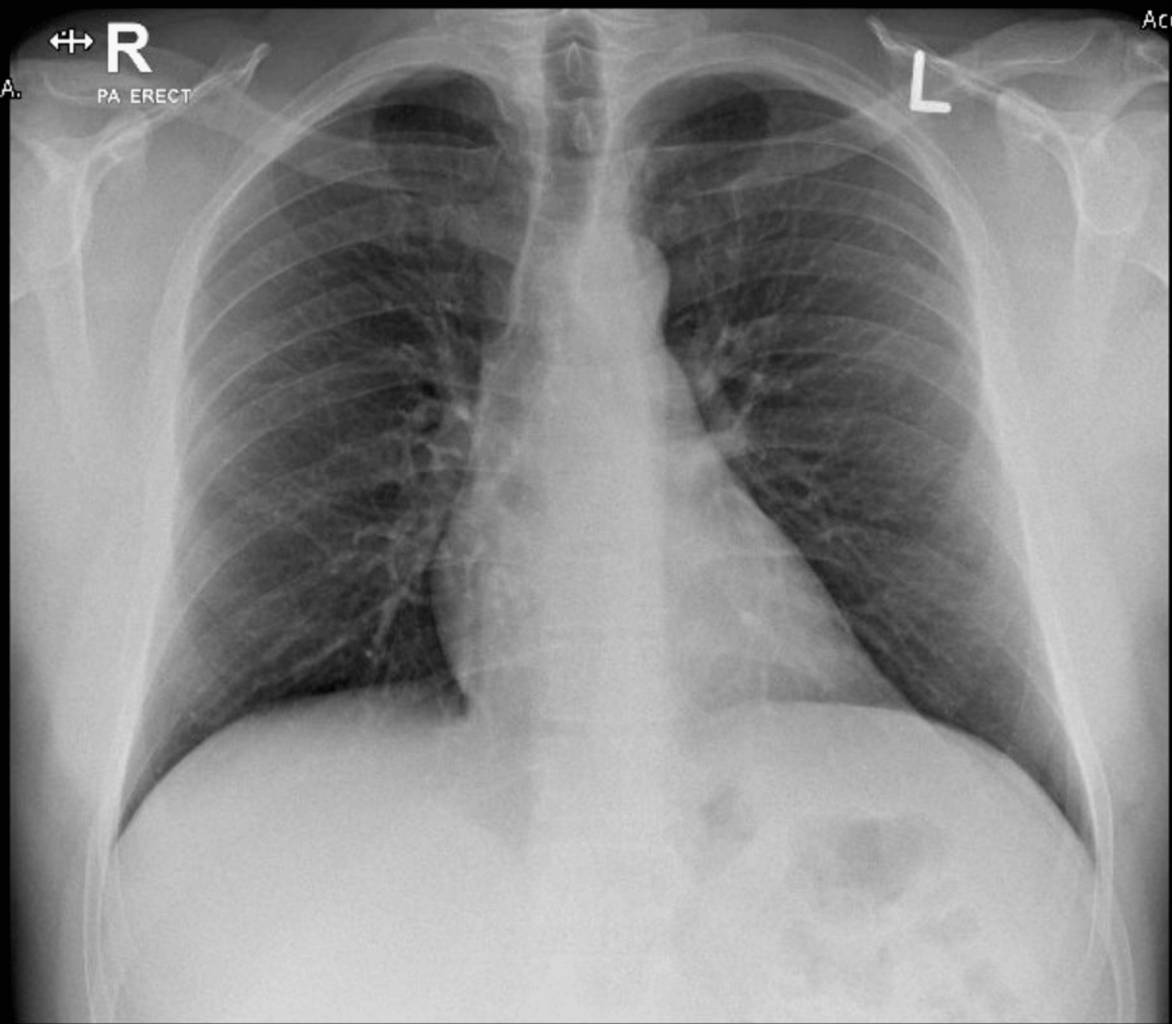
Chest X-ray Posterior-anterior chest radiograph demonstrating clear lung fields with no evidence of pulmonary congestion, consolidation, or pleural effusion.
